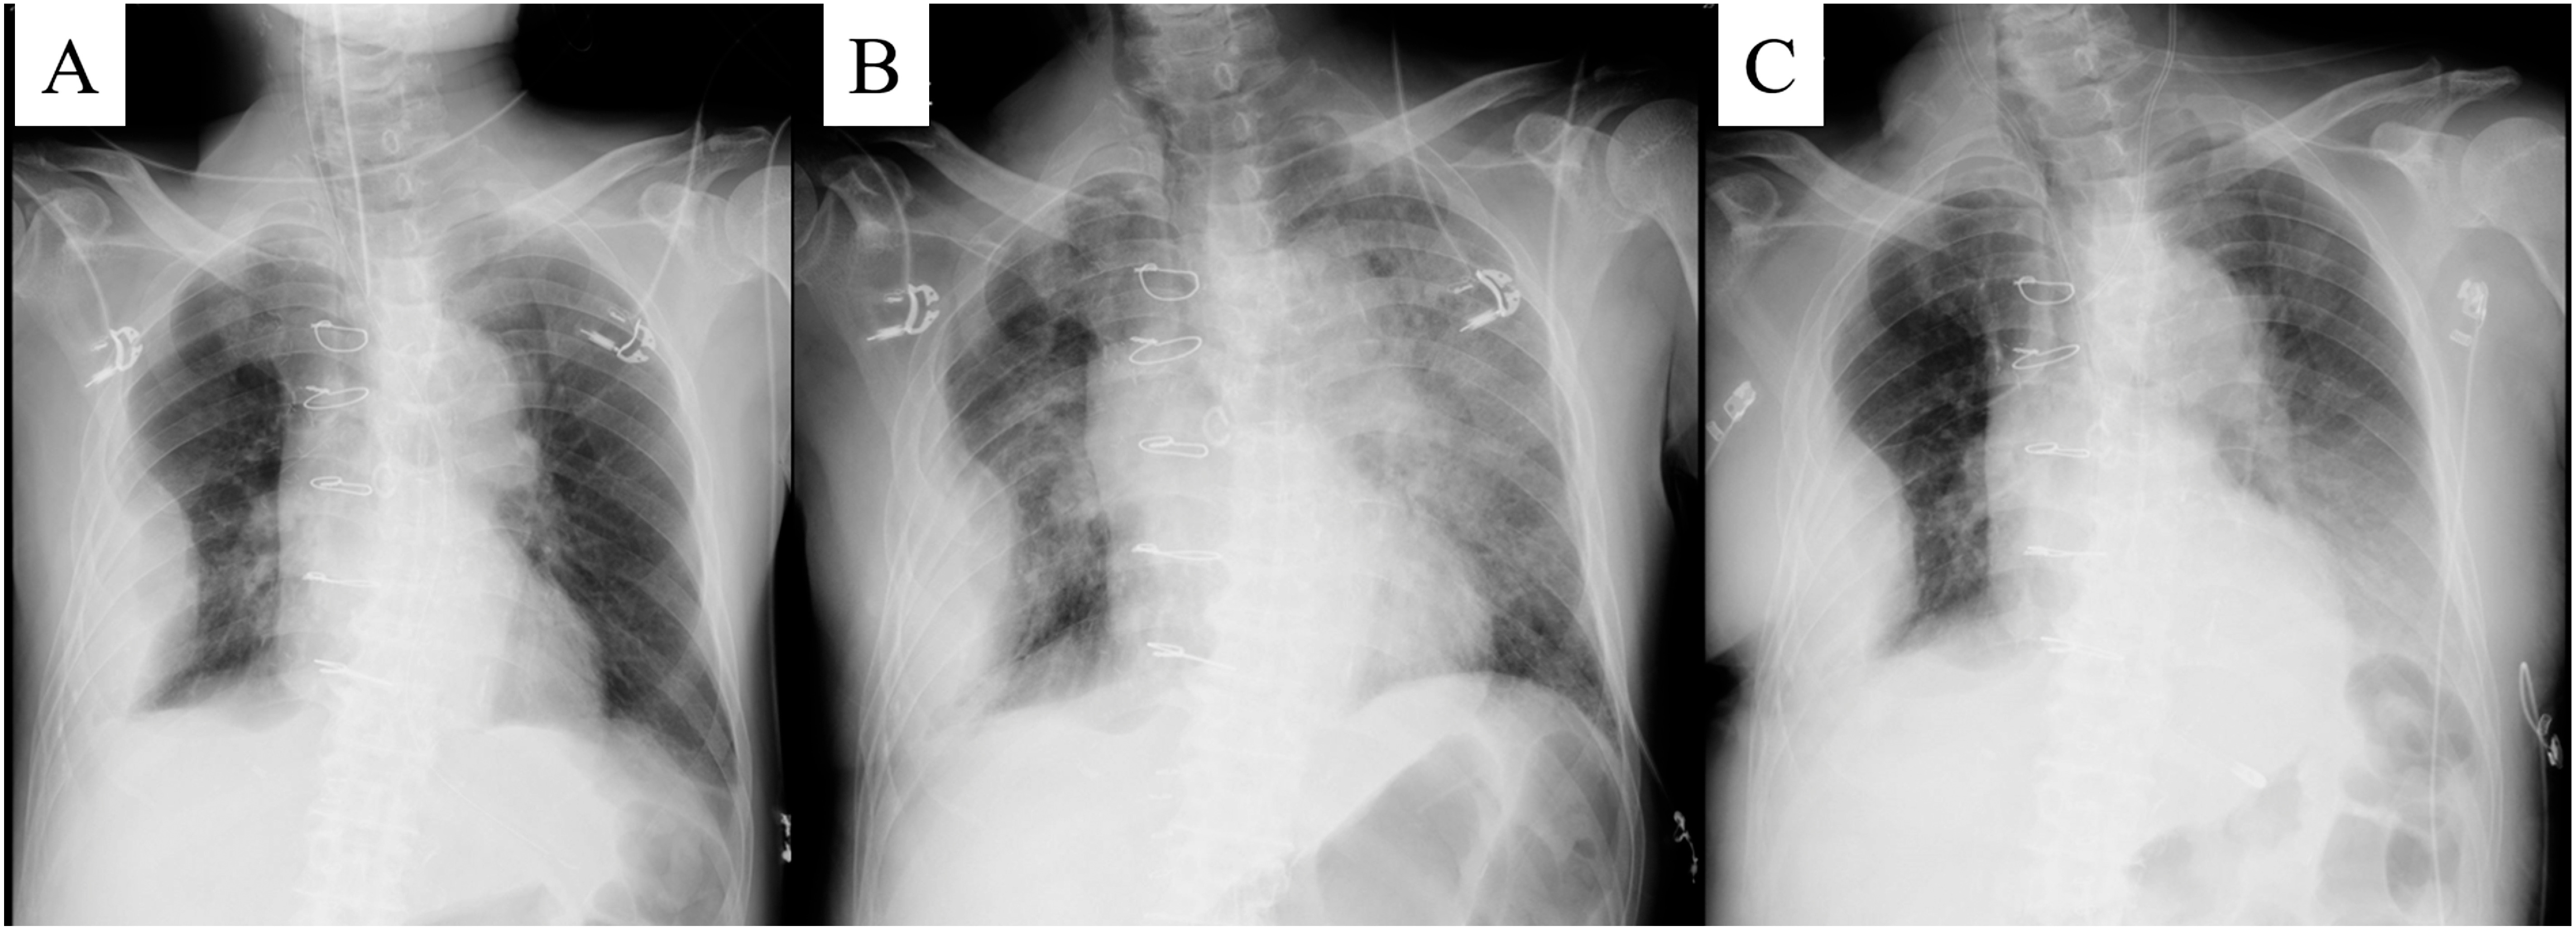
Fig. 2 Postoperative chest X-rays showing longitudinal changes.
